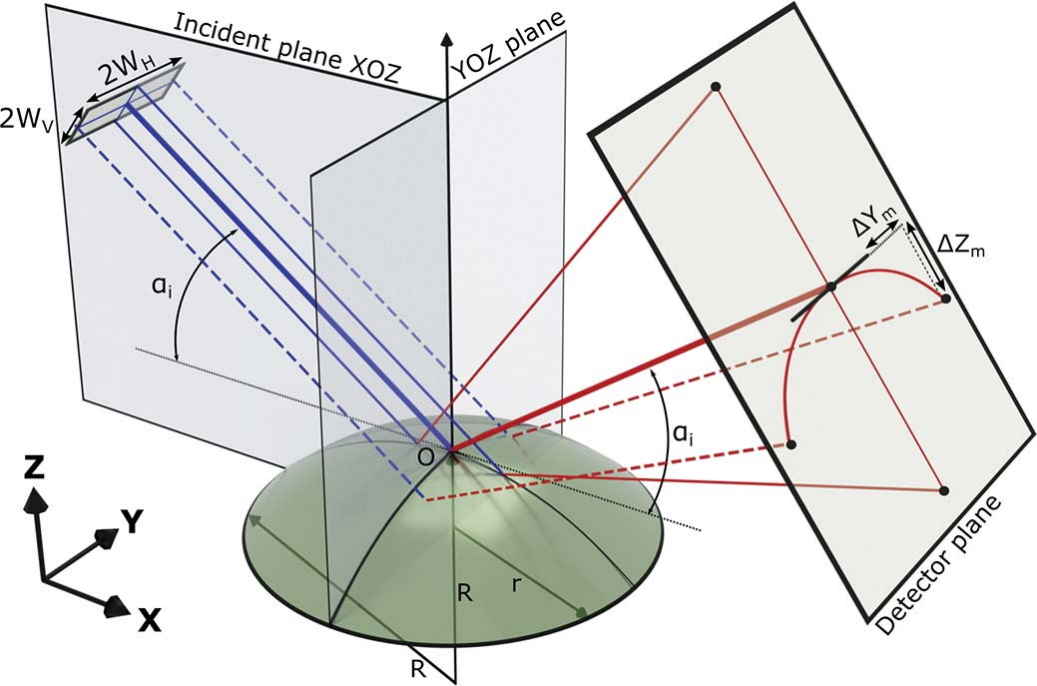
Scheme of the incidence and reflected X-ray beam on a spherical surface with a coordinate system selection. Solid blue and solid red rays represent the primary and reflected rays propagating in the incident plane *XOZ*, while dashed blue and red rays are in the orthogonal plane. A curved surface is considered as a cut from a sphere of radius *R* having base radius *r*. Δ*Y*
